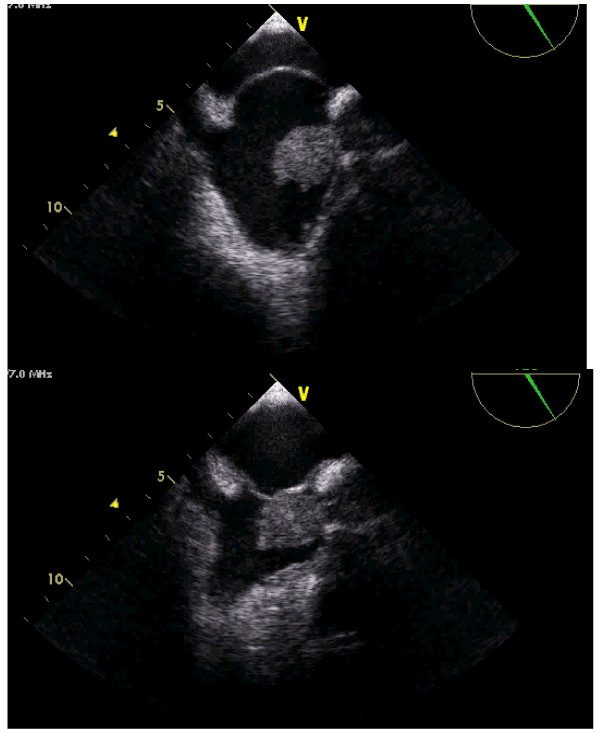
Transesophageal echocardiography. Playing games with a thrombus: a dangerous match. The hyperdinamic ASA beats the mass in every cardiac cycle, like a racket beating a ball in a ping-pong match.
